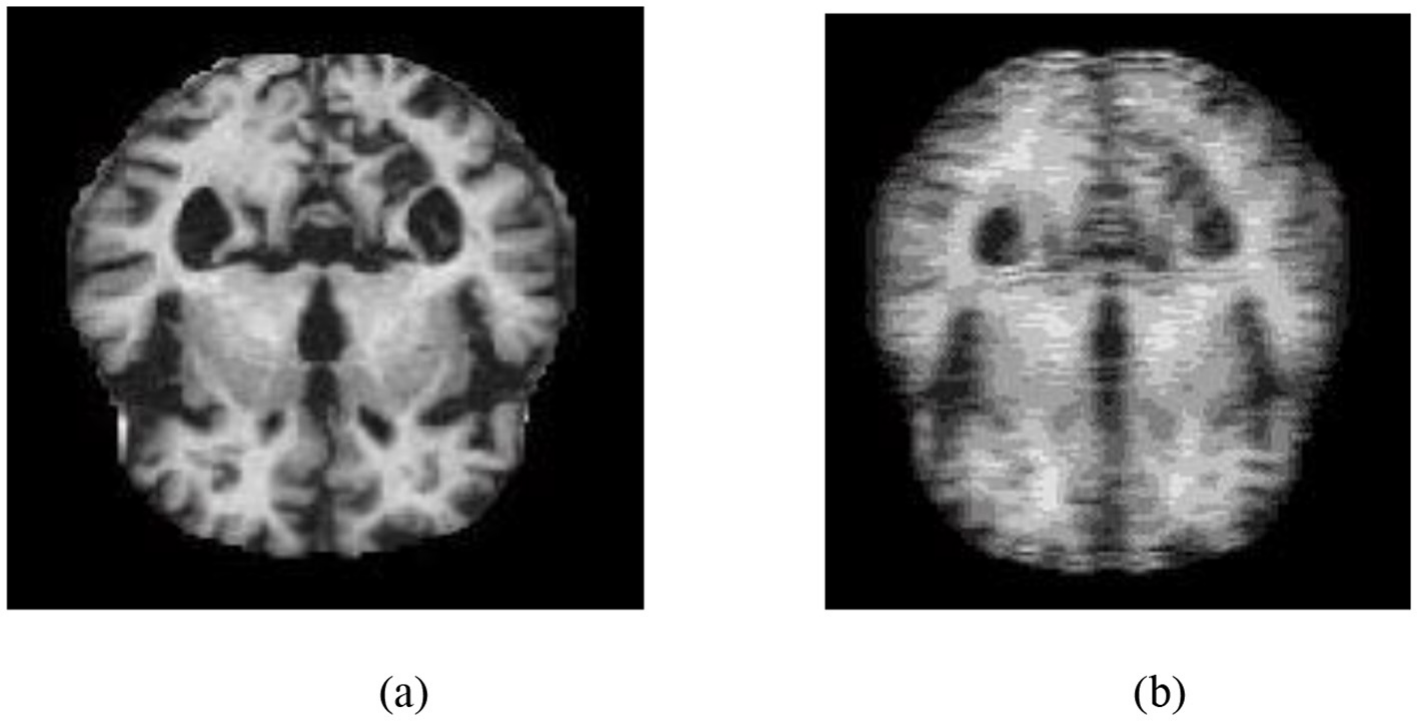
(a) T1-weighted sMRI image of an AD subject. (b) Corresponding Gabor-transformed image highlighting texture and frequency-domain features.
